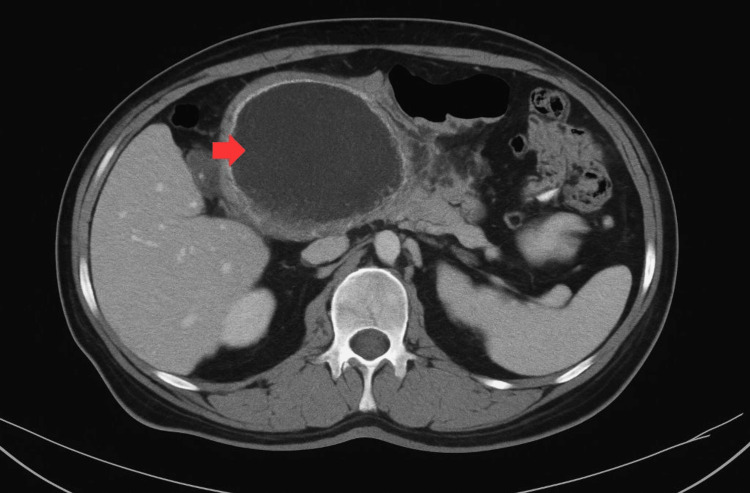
Contrast-enhanced abdominal CT scan showing evidence of a pancreatic collection (red arrow).
